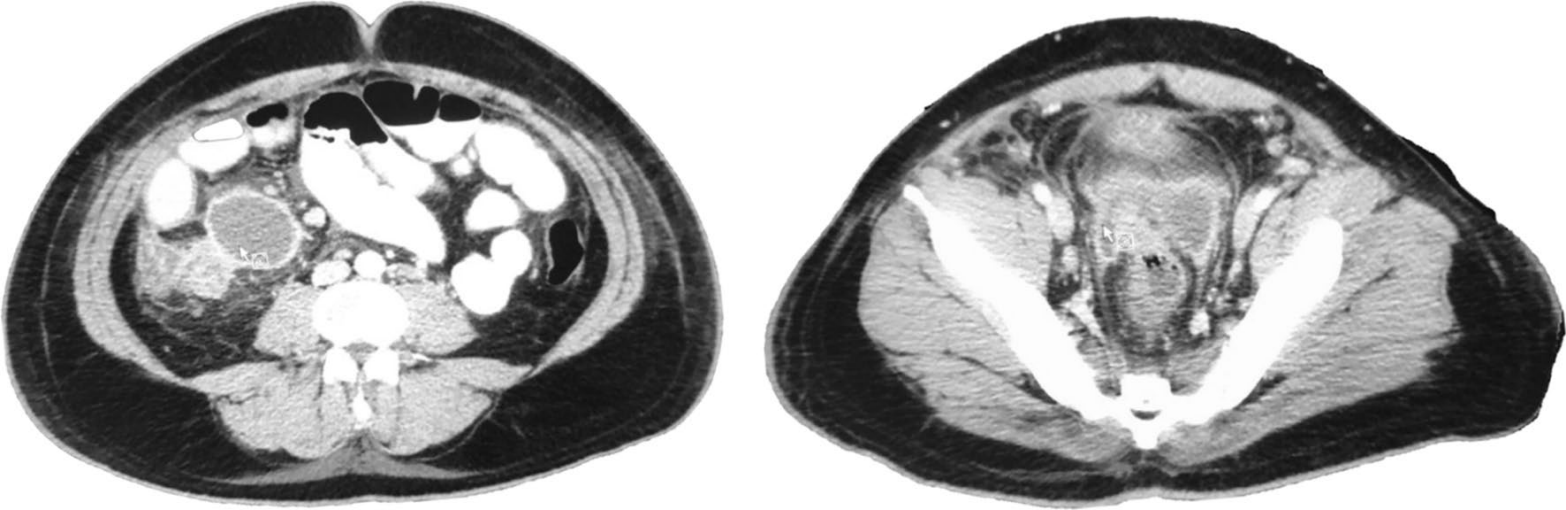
Abdomino-pelvic CT. Interloop edema, perivesical fluid infiltration and scant free fluid in the abdomino-pelvic cavity
